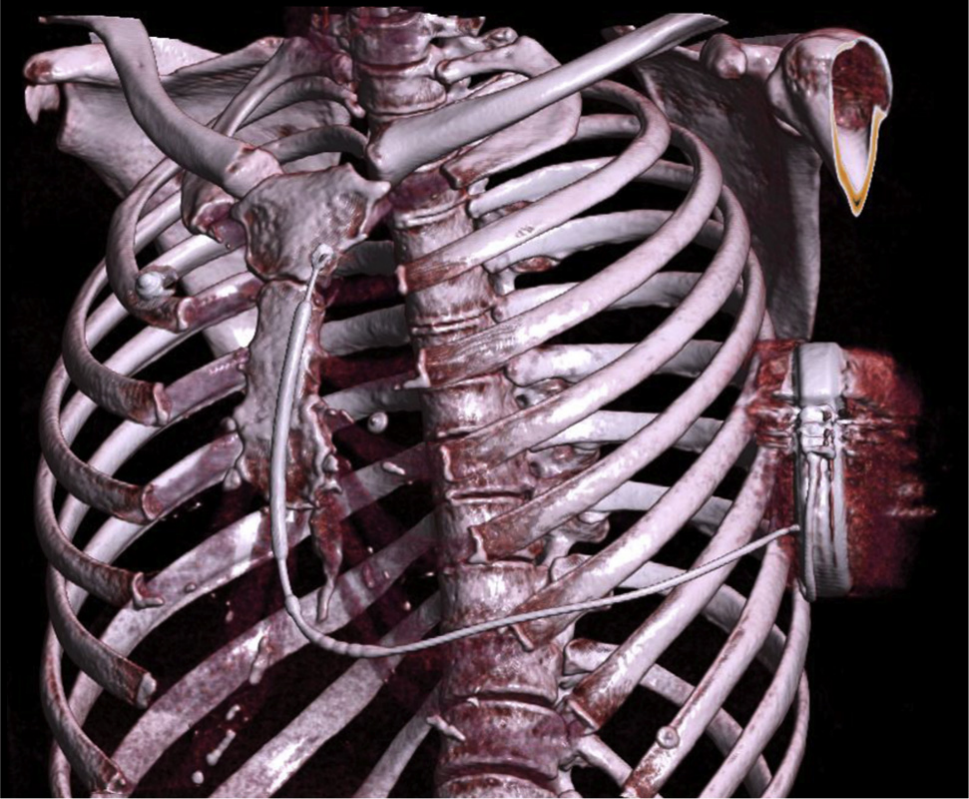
Chest computed tomography with volume rendering showing the repositioned subcutaneous implantable cardioverter-defibrillator lead in correct position within the subcutaneous tissue.
